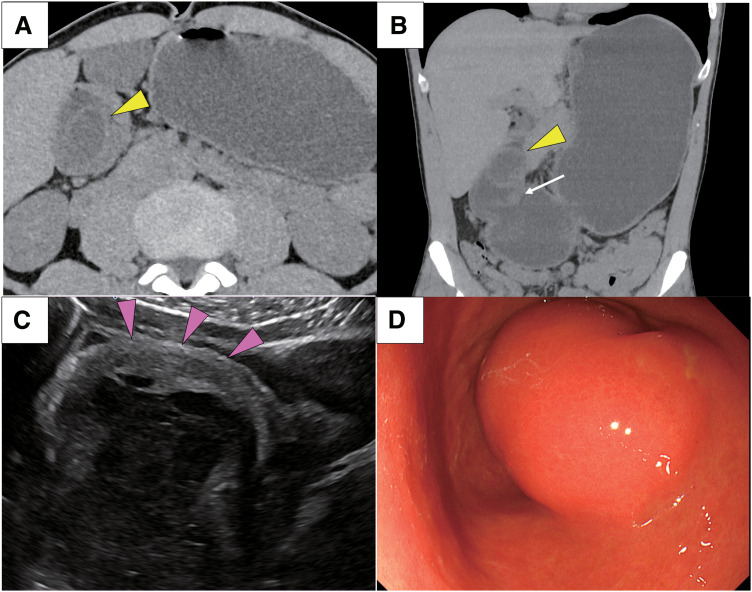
Images of preoperative examinations. (A, B) Abdominal computed tomography depicted a 40-mm low-density mass that extended beyond the pyloric ring (white arrow) and descended into the duodenal bulb, showing a target sign (yellow arrowhead). (C) Ultrasonography depicted a 40-mm mass with fluid accumulation arising from the gastric submucosa (magenta arrowheads). (D) Gastric fiber revealed a submucosal lesion on the posterior wall of the gastric pylorus.
